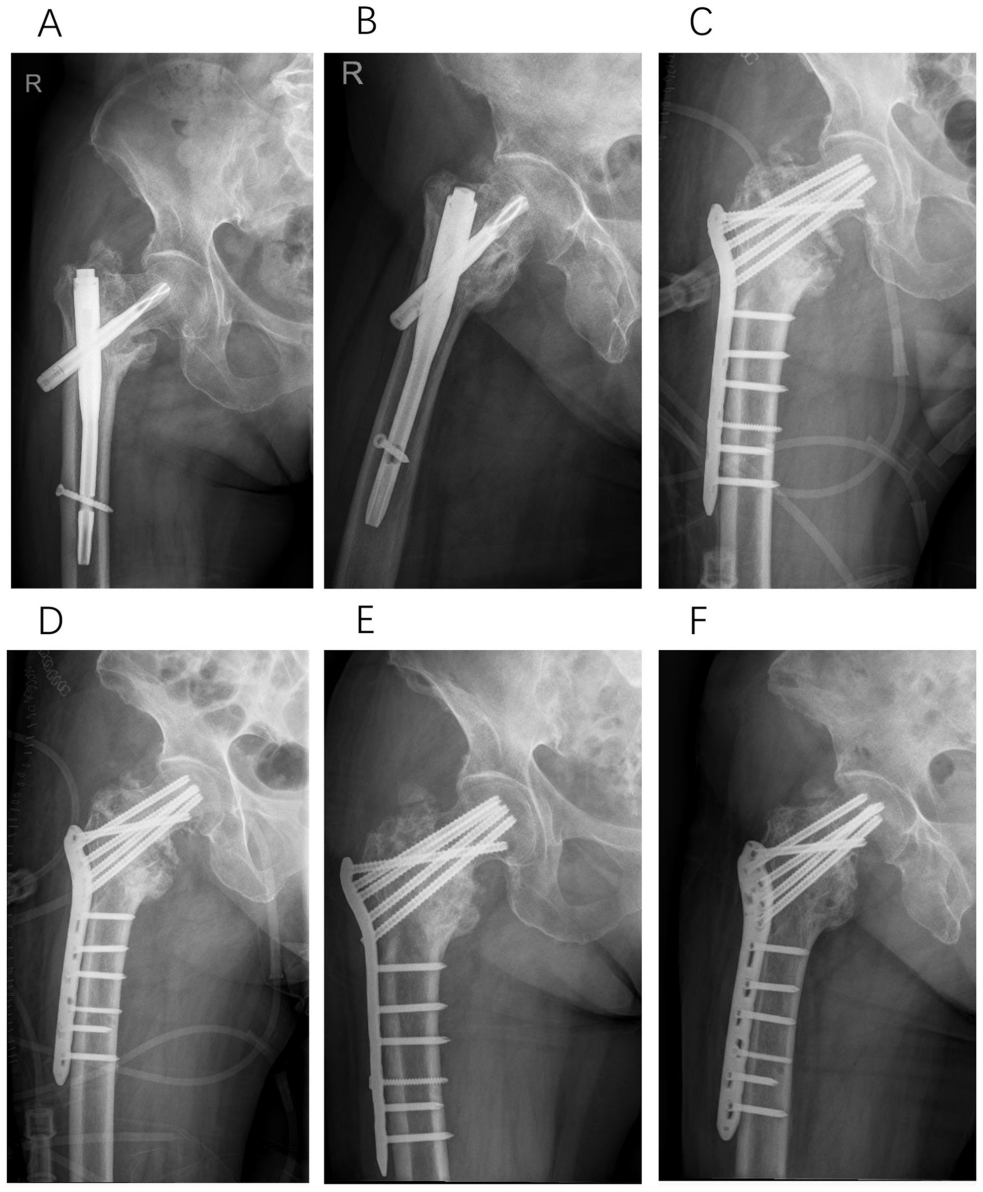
Type II-B, a 69-year-old male patient underwent PFNA fixation for a right intertrochanteric femoral fracture. Twelve months post-operatively, radiographic evaluation revealed coxa vara and non-union at the fracture site. In the subsequent revision surgery, we addressed the varus deformity through corrective osteotomy, performed structural bone grafting, and implemented plate internal fixation. Successful bony union was achieved 5 months following this revision. (A, B) Anteroposterior and lateral X-rays of the femur obtained 12 months after initial PFNA fixation. (C, D) Anteroposterior and lateral X-rays of the femur immediately following the revision surgery. (E, F) Successful bony union confirmed 5 months post-revision surgery.
